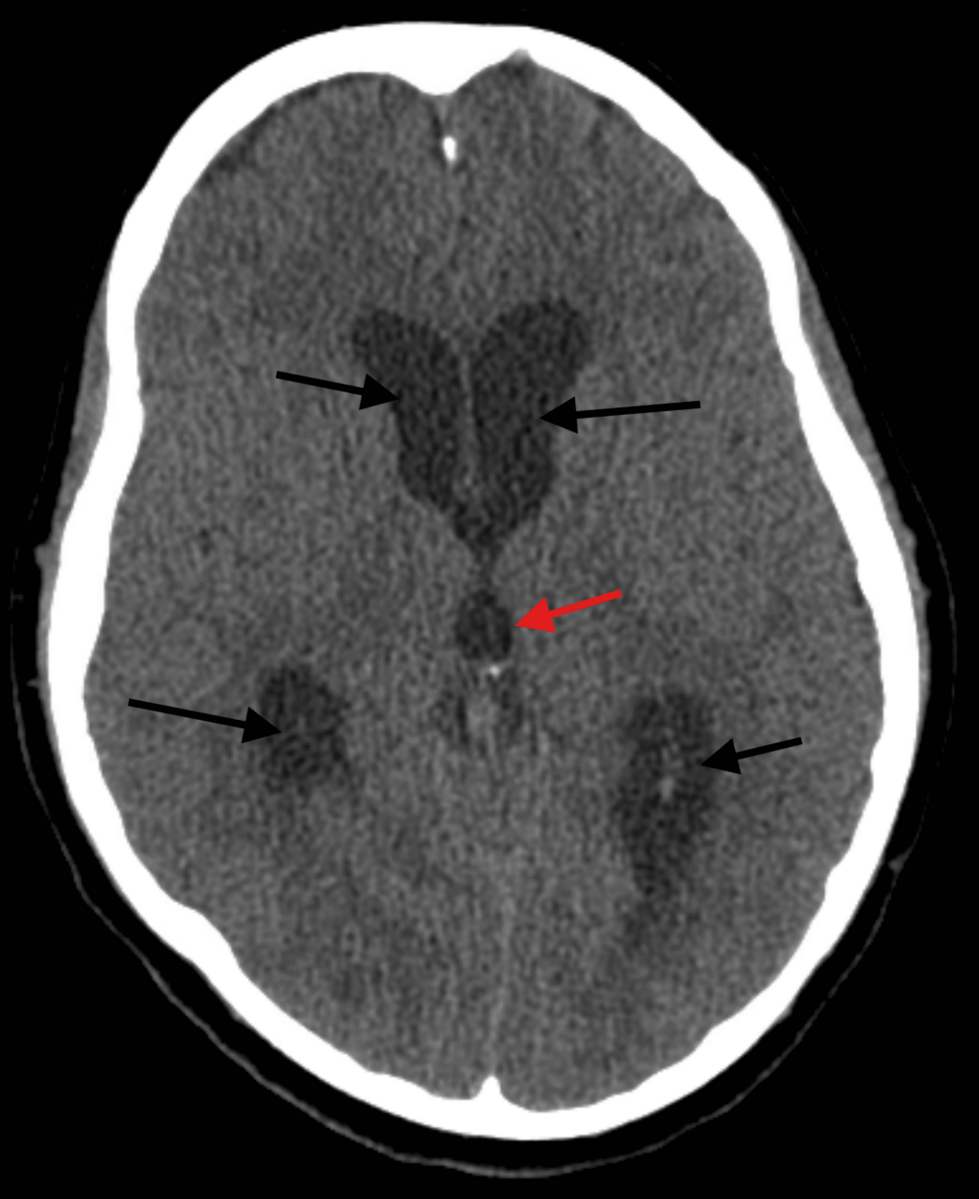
CT head without contrast showing communicating hydrocephalus with dilated lateral ventricles (black arrows) and third ventricle (red arrow)
